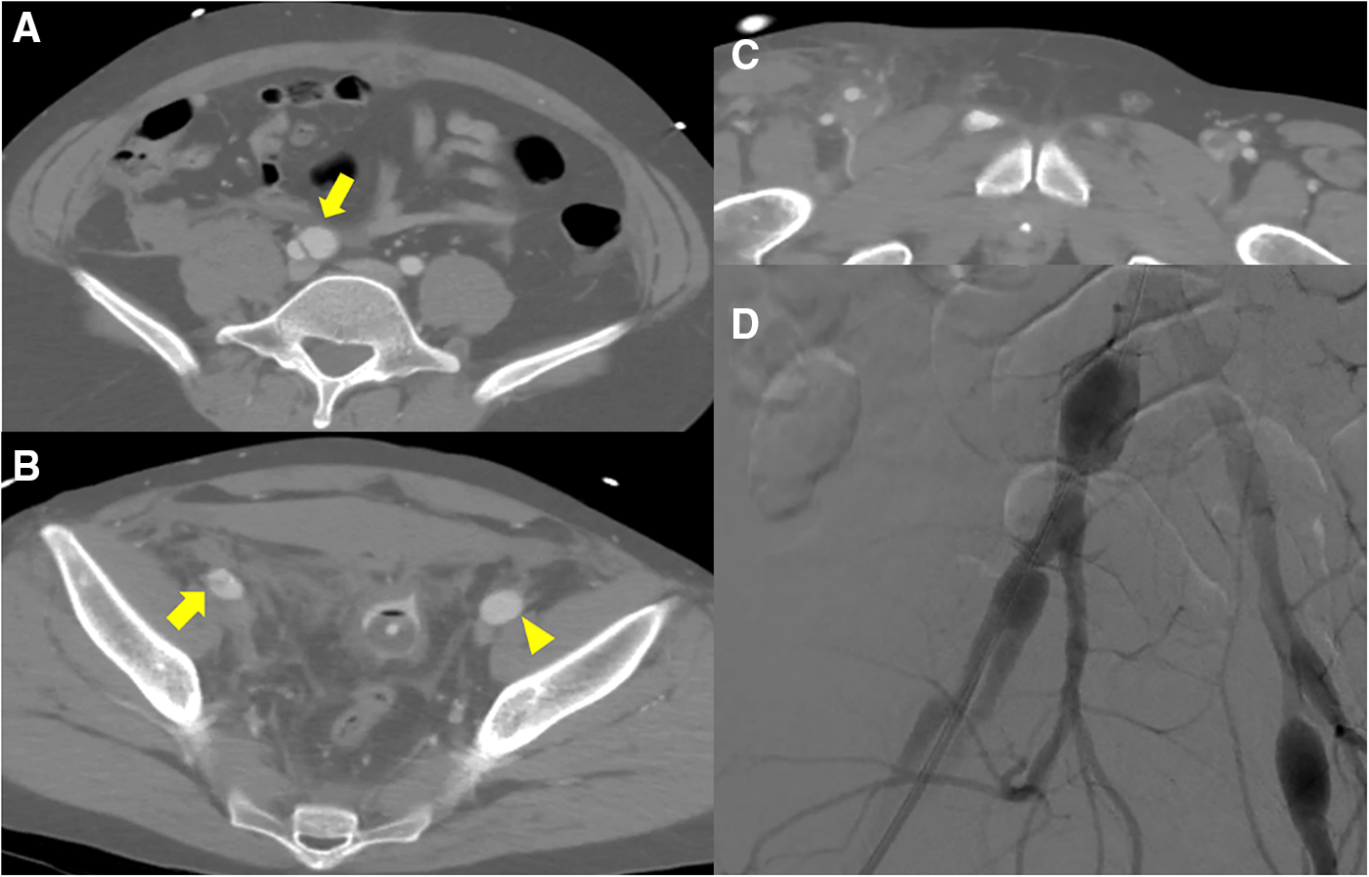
Initial computed tomography scan shows (A) dissecting aneurysms (*yellow arrow*) from right common iliac artery to (B) external iliac artery and aneurysmal dilatation of left external iliac artery (*yellow arrowhead*). (B, C) Hemoperitoneum was detected in the pelvic cavity and the area surrounding right superficial femoral artery; however, no active bleeding was detected. (D) Intraoperative fluoroscopic angiography shows pathological aneurysms on both sides.
